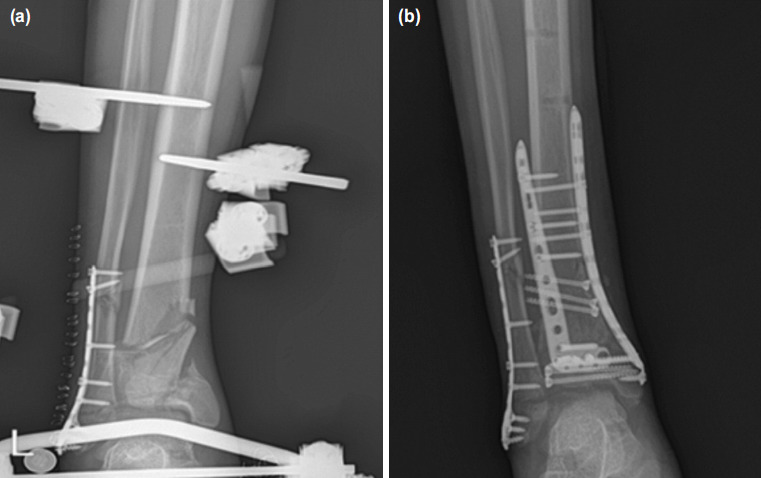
(a) Radiograph images of the patient who underwent fibula fixation in the first stage. Fracture of the fibula was treated with open reduction internal fixation in the first stage. (b) Definitive treatment was applied to the fracture of the tibia after soft tissue swelling resolves.
